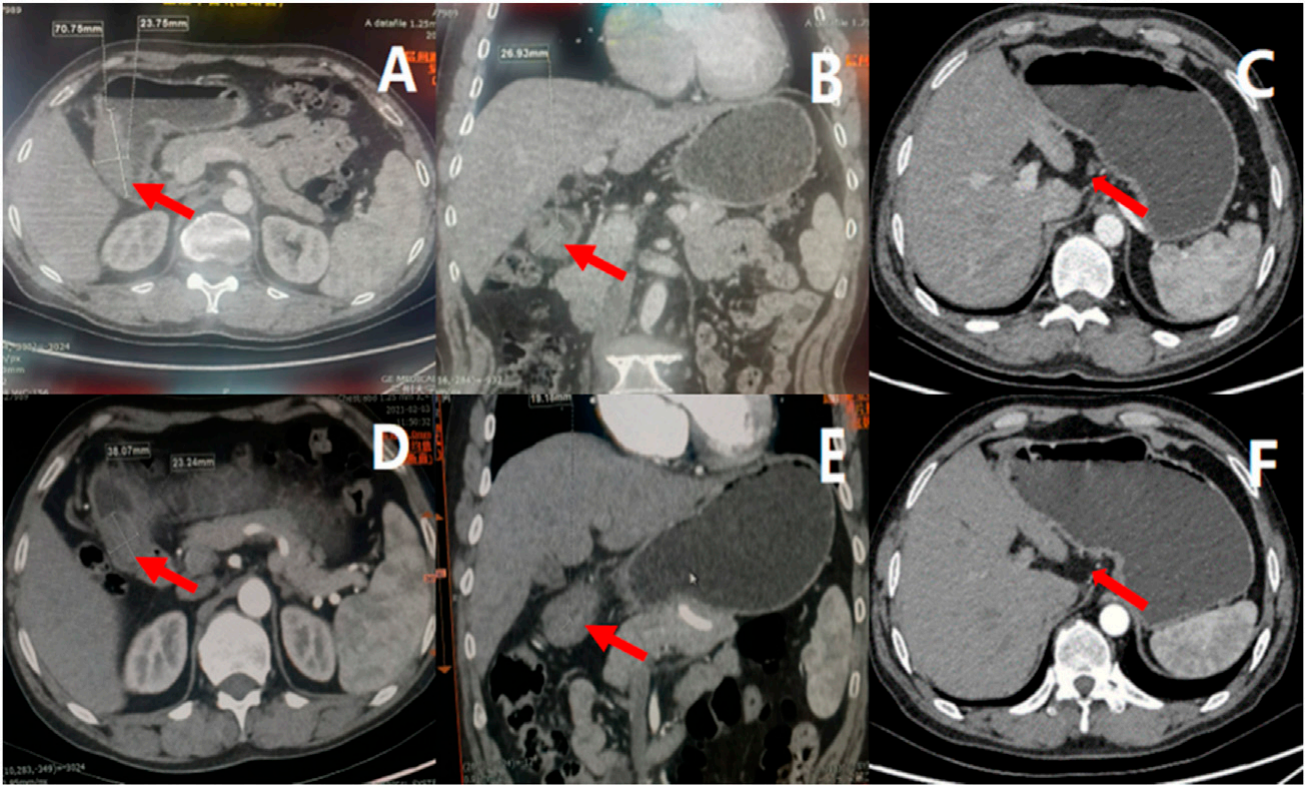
Results of enhanced CT before and after neoadjuvant therapy, indicated tumor and lymph node volume decreased after three cycles of treatment. (A) showed the cross section of gastric antrum tumor before treatment, (B) showed the coronal view of gastric antrum tumor before treatment, (C) showed the enlarged lymph nodes before treatment; (D) showed the cross section of gastric antrum tumor after neoadjuvant treatment, (E) showed the coronal view of gastric antrum tumor after neoadjuvant treatment, (F) showed the enlarged lymph nodes after neoadjuvant treatment.
